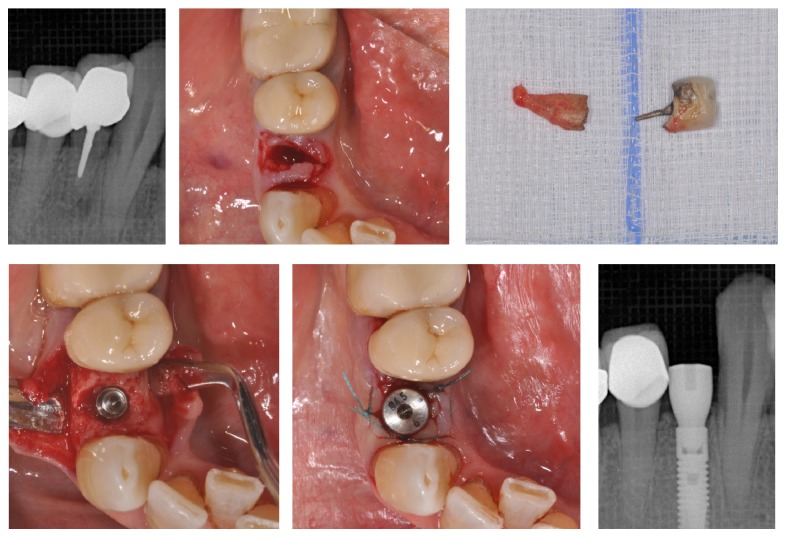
Type 1 implant positioning with transmucosal healing, surgical and radiographic view.
